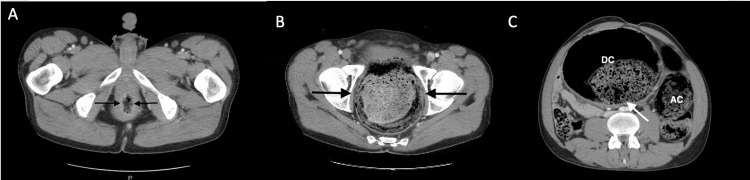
AHD with the aganglionic segment of the lower part of the rectum in a 33-year-old man. (a,b) Contrast-enhanced transverse CT scans show markedly dilated feces: filled proximal upper part of the rectum and the rectosigmoid junction (arrows in b) with a transition zone and a distal narrowed rectum (arrows in a). The distal rectum also appears to also have a thickened wall. (c) Contrast-enhanced transverse CT scans (b obtained at lower level than c) obtained in a 33-year-old man with AHD show markedly dilated feces: filled AC and DC with sigmoid volvulus. AHD: adult Hirschsprung's disease; CT: computed tomography; AC: ascending colon; DC: descending colon
